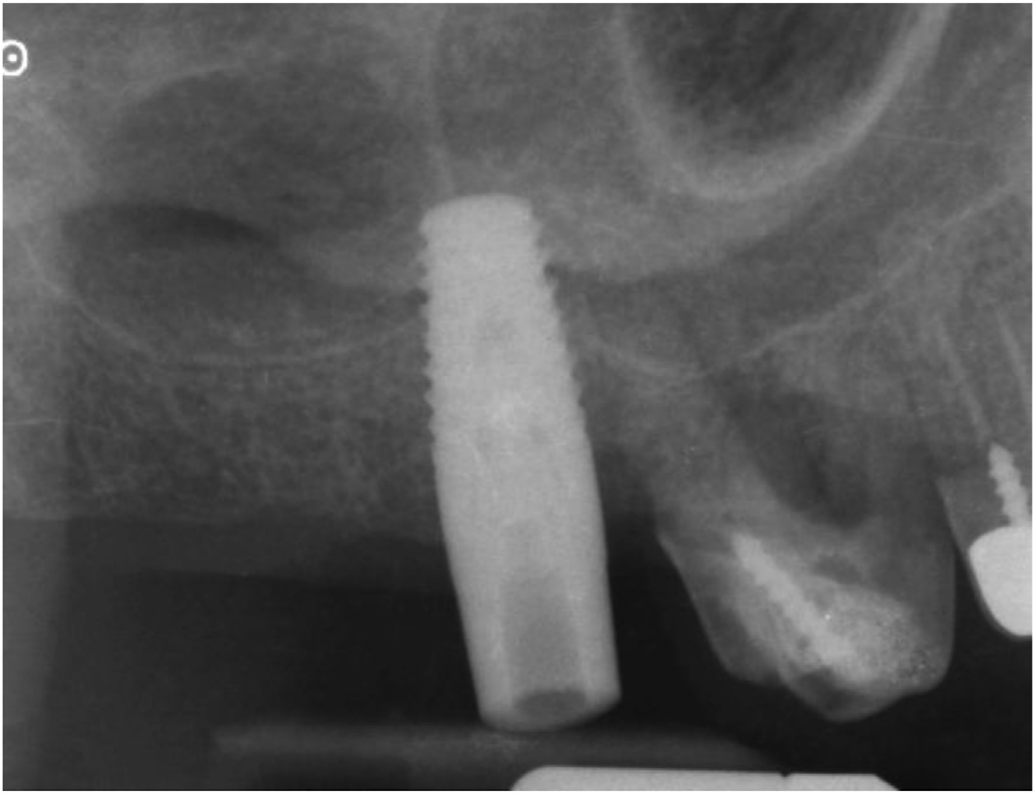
Periapical X-ray taken with the paralleling technique of the clinical situation shown in figure 6, immediately after implant insertion. When compared with Figure 5, it is clear that the implant tip is above the original sinus floor and that is surrounded by some shifted bone structure.
